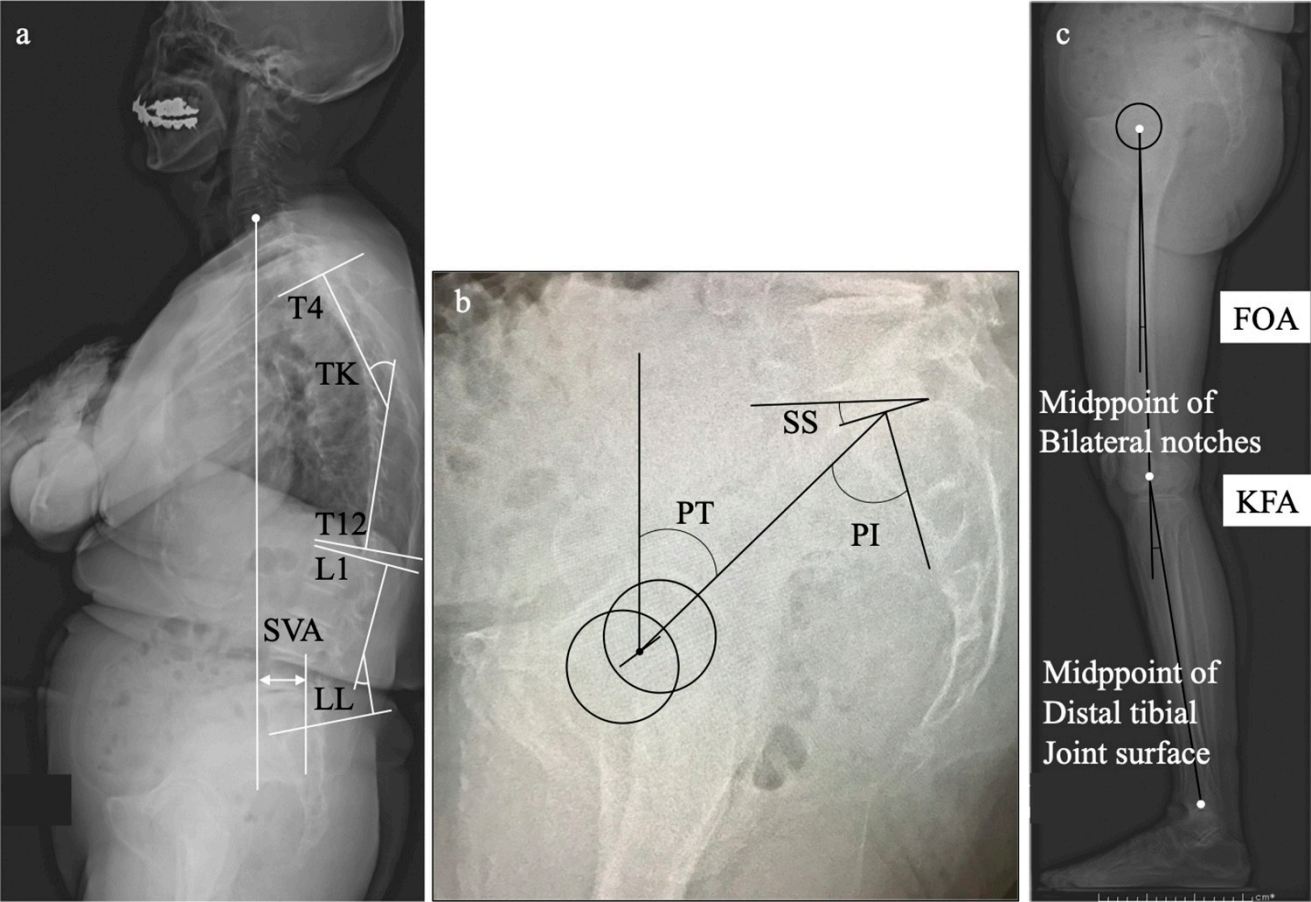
Sagittal alignment parameters in the standing position. Spinal parameters, TK (thoracic kyphosis), LL (lumbar lordosis), and the SVA (sagittal vertical axis), were measured on a lateral whole-spine radiograph (a). Pelvic parameters, the PT (pelvic tilt), SS (sacral slope), and PI (pelvic incidence), were measured (b). Lower limb parameters, the FOA (femur obliquity angle) and KFA (knee flexion angle), were measured (c).
